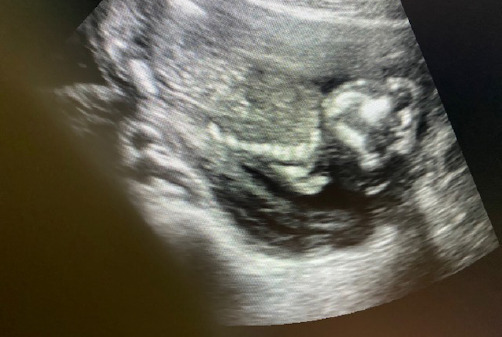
Ultrasound images showing the absent calvaria and exposed brain tissue.
